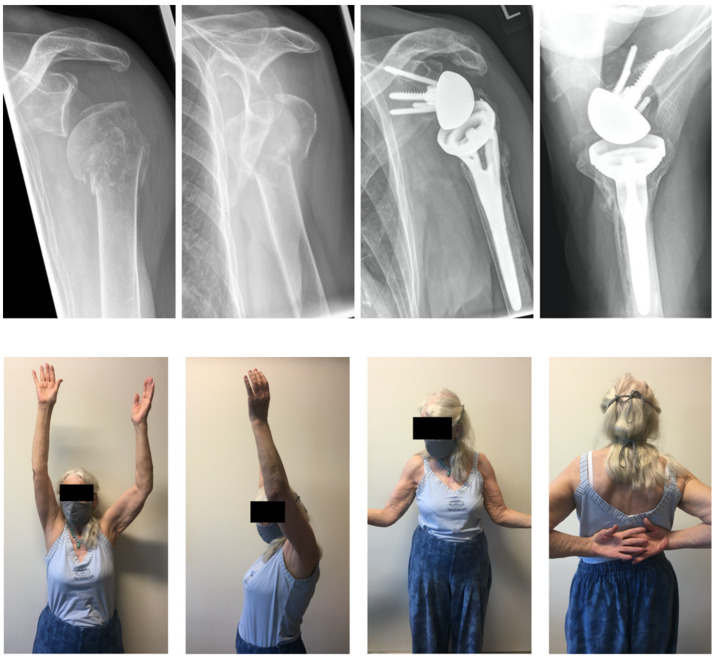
This 77-year-old woman sustained a type III fracture in preoperative radiographs (upper two left) after a fall onto her left shoulder while hiking. At 50 months post-RSA, the patient was very satisfied with excellent function (lower bottom) and a CS of 81 points, a relative CS of 99%, and a SSV of 95%. Both the greater and lesser tuberosities show healing and no scapular notching is visible on post-op (upper two right) images.
